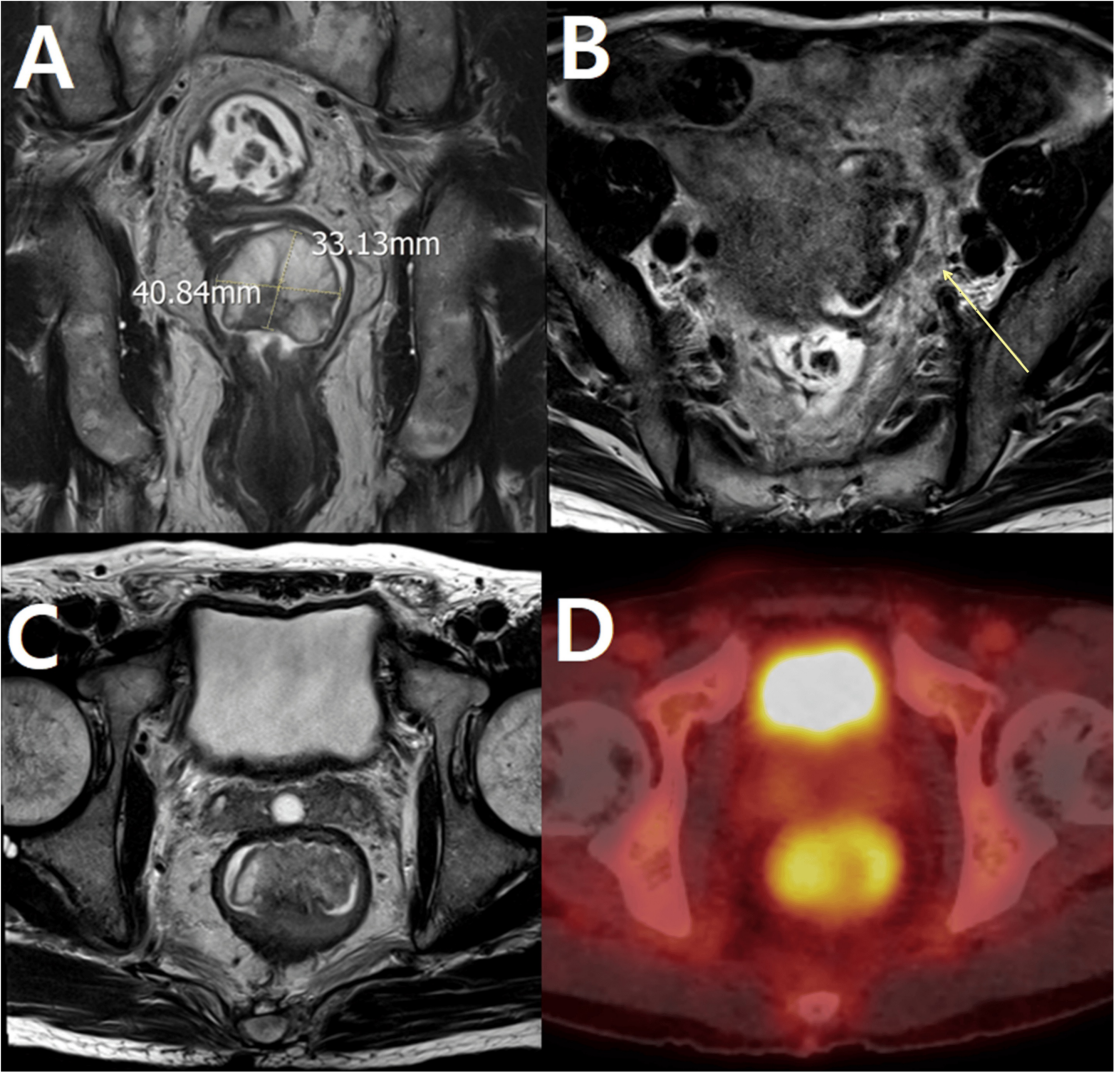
MRI and PET/CT findings. About 4.1 × 3.3 cm pedunculated mass with internal T2 high signal intensity (SI) in the posterior wall of the mid-rectum (4-8 hour direction) (A) with a possible pathological lymph node (arrowed) around the peri-rectum (B). Hypermetabolism is observed on PET imaging at the location of the enhancing mass seen on MRI (C and D). MRI, magnetic resonance imaging; PET, positron emission tomography PET, positron emission tomography
